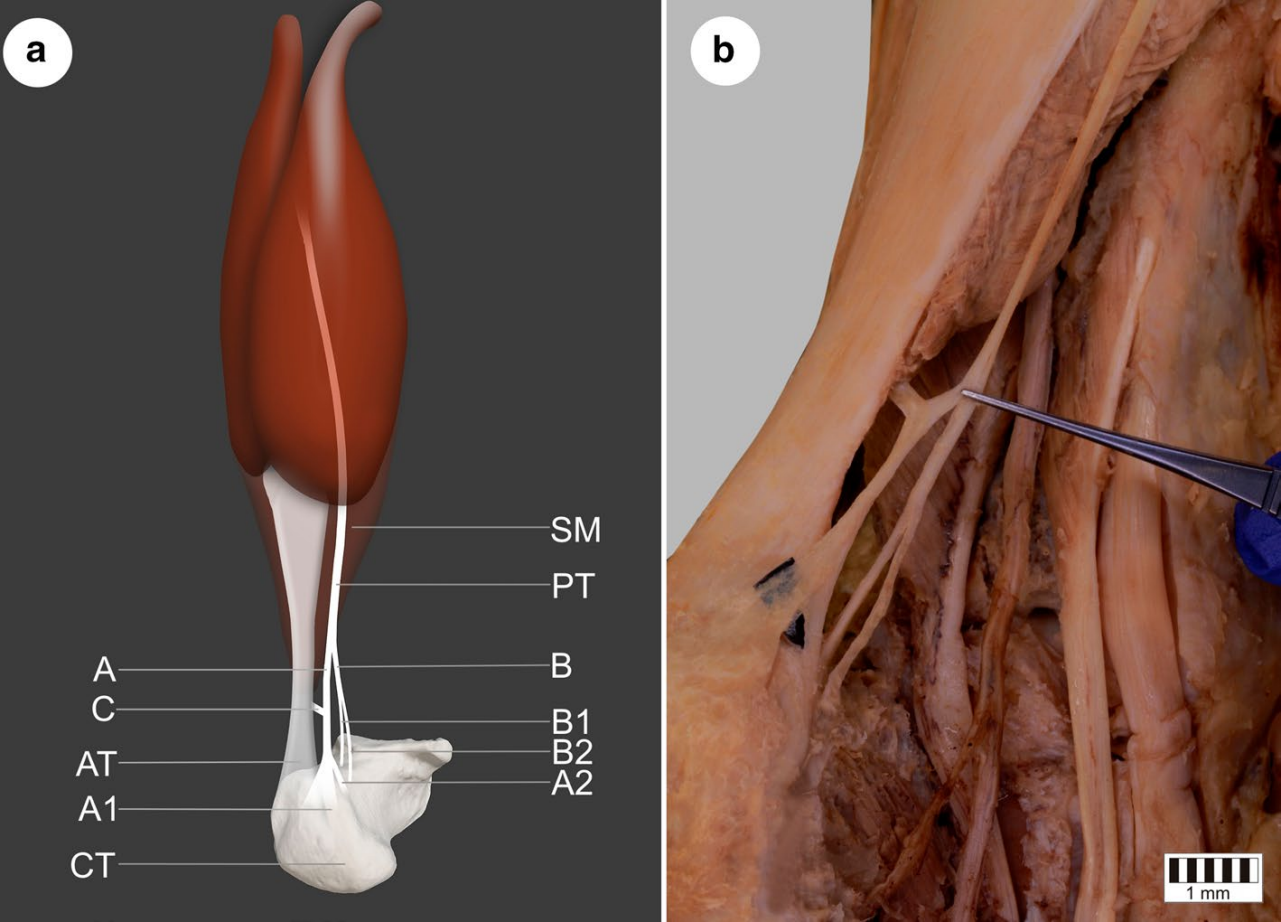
a The presented variant of the plantaris tendon insertion: a posteromedial view of the left crural and foot region. b The Type VII of plantaris tendon insertion; *SM* soleus muscle, *PT* plantaris tendon, *AT* Achilles tendon, *CT* calcaneal tuberosity, *A, A1, A2, B, B1, B2, C* specific bands of the plantaris tendon (Type VII); the solid line surrounds the superior surface of the calcaneus; the dashed line surrounds the medial surface of the calcaneus
